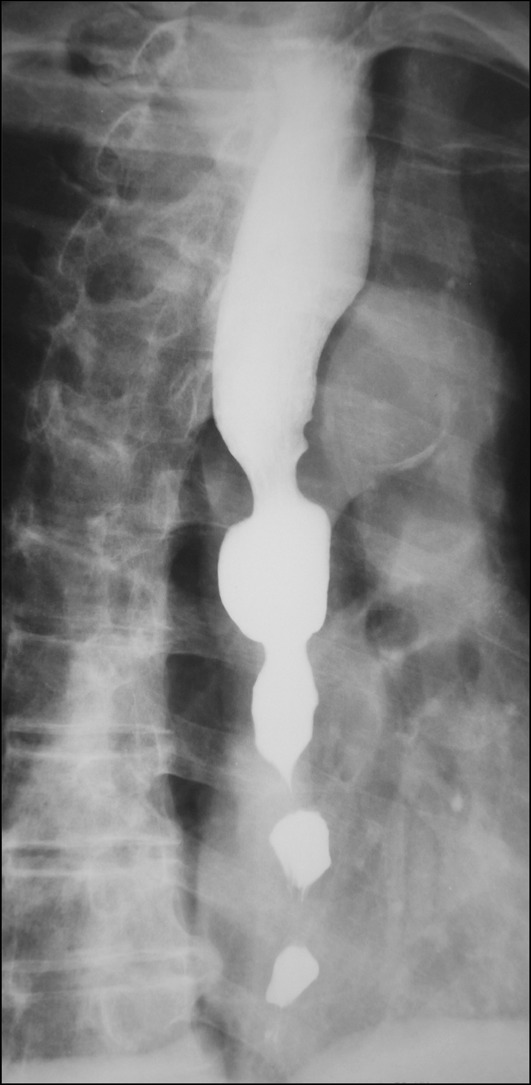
Radiographic appearance in a patient with DES
